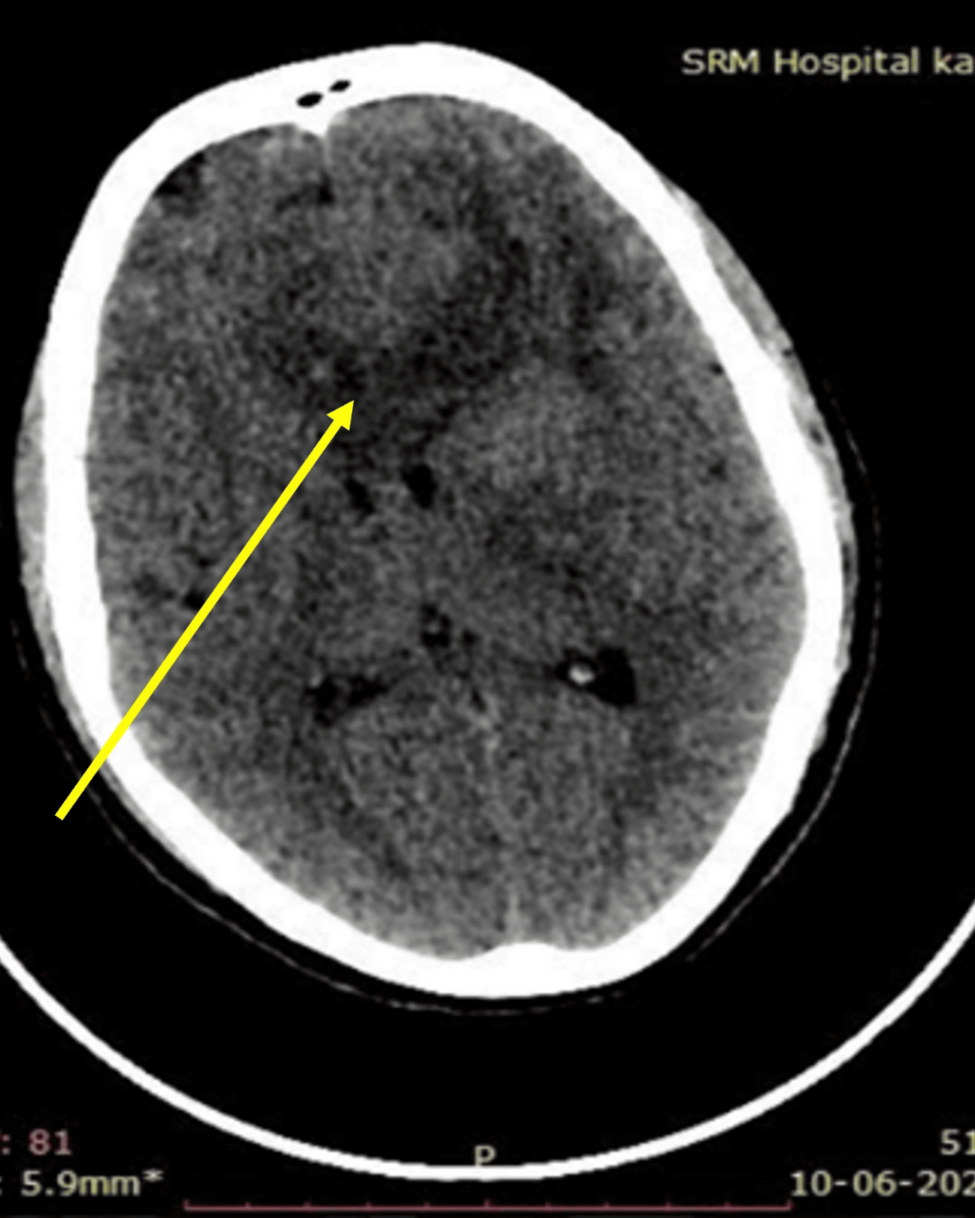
CT brain showing hypodense areas in the bilateral frontal and basifrontal regions, more pronounced on the left side. The hypodense areas extend to the rostrum and body of the corpus callosum on the left side, causing mass effect and midline shift.
